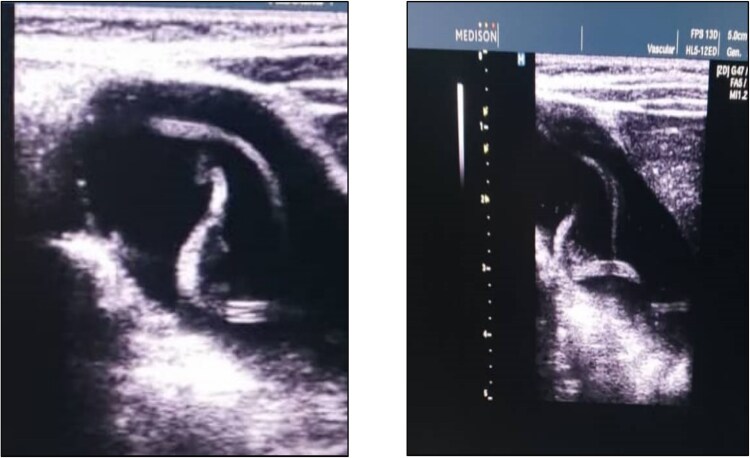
Ultrasound image of the patient shows *Ascaris* worm in gallbladder.
